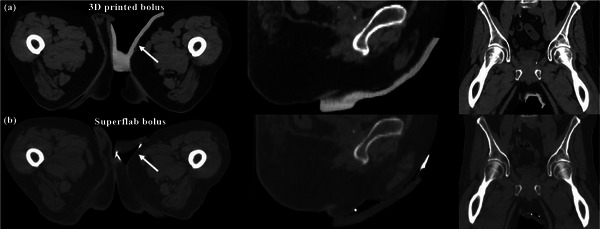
CT images of (a) a 3D‐printed bolus and (b) a Superflab bolus for the same patient. From left to right: axial, sagittal, and coronal views. CT, computed tomography.
